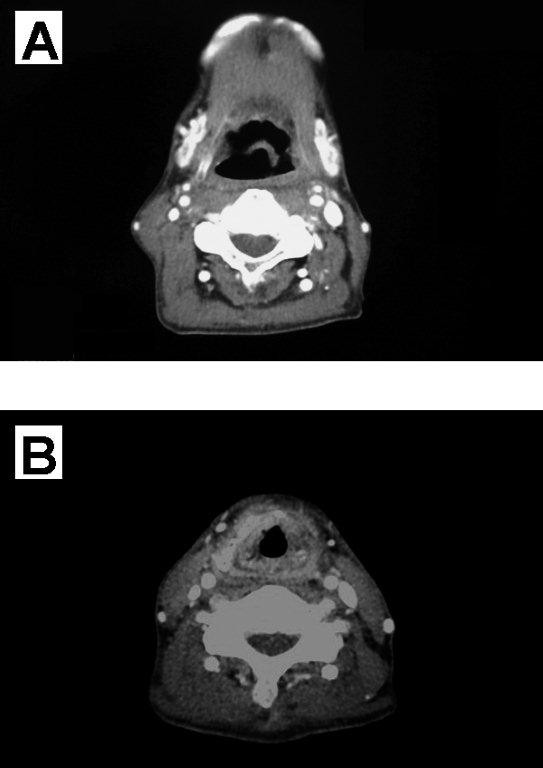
CT results after administration of steroids and antibiotics. These show the disappearance of space-occupying lesions initially suspected as tumor recurrence at the levels of the (a) epiglottis and (b) thyroid cartilage.
